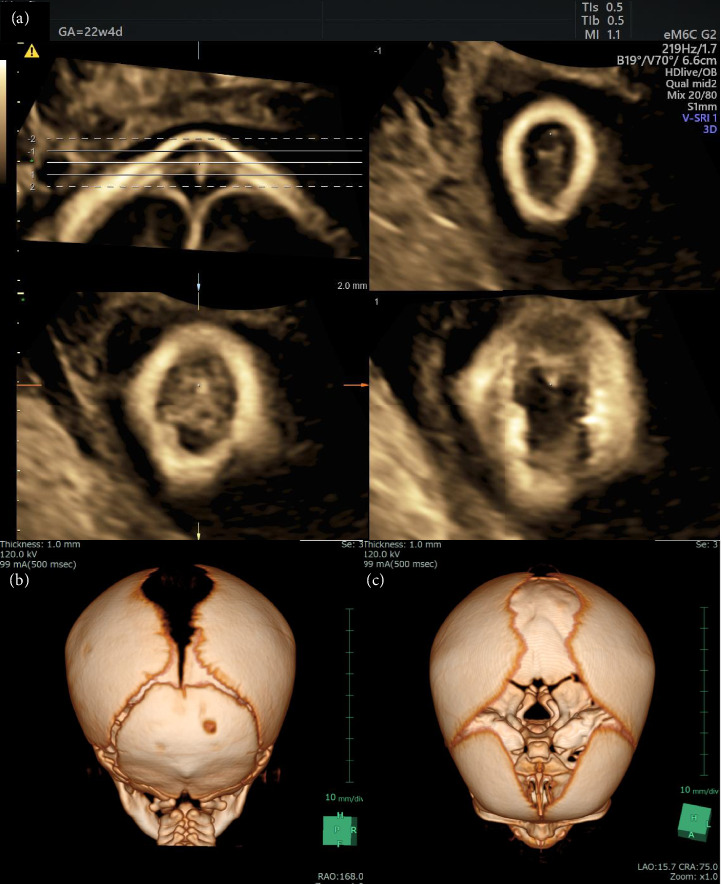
Images obtained during volume contrast imaging (VCI) of the head at 22 weeks of gestation and during postnatal computed tomography (CT). (a) The circular defect of the fetal skull bone, connecting to the sagittal suture in the right lower image, can be seen. (b) The posterior view of postnatal CT image showing the posterior fontanelle and skull bone defect of the neonate. (c) The parietal view of postnatal CT image showing the anterior fontanelle and skull bone defect of the neonate.
